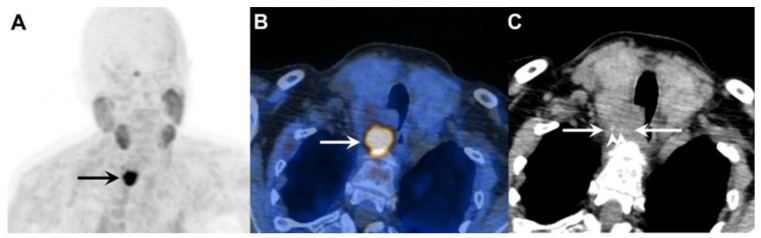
A 73-year-old woman with primary hyperparathyroidism due to parathyroid carcinoma. Legend: (A) Coronal [^18^F]fluorocholine PET maximum intensity projection (MIP) image demonstrates an intensely tracer-avid lesion in the neck (black arrow, SUV_max_ 18.9). (B) Axial fused [^18^F]fluorocholine PET/CT image shows the tumor (white arrow) located deep to the right lobe of the enlarged thyroid gland. (C) On the corresponding unenhanced CT image, there is loss of the fat plane between the tumor (white arrows) and surrounding structures, with intralesional stippled calcifications visible (white arrowheads).
